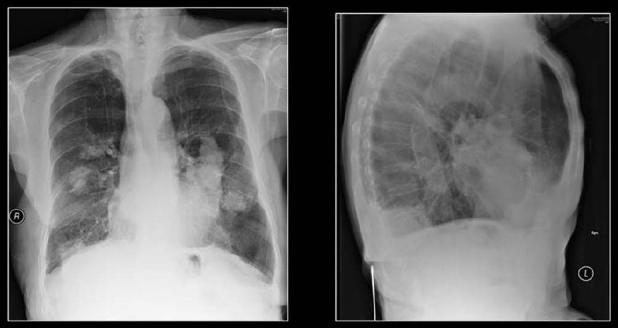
PA and lateral chest radiographs showing multiple high-density lung masses in a patient with a nonmucinous adenocarcinoma of the sigmoid colon (see CT scans in Figure 21)
